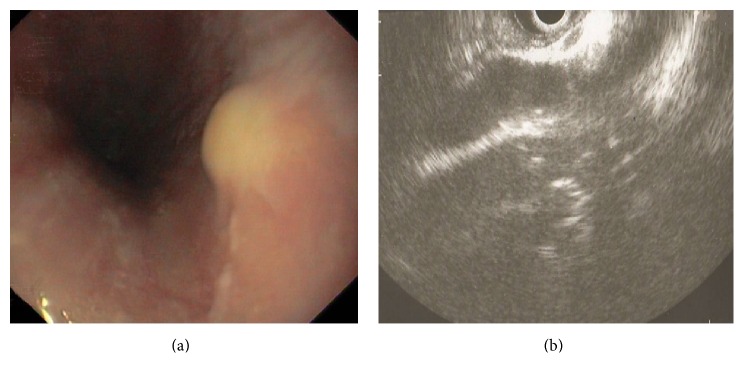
Endoscopic view of an elevated yellow submucosal lesion in esophagus (a). Endosonographic image showed a nonhomogeneous hyperechoic submucosal lesion with a diameter of 12 mm and without extension to muscularis layer (b).
